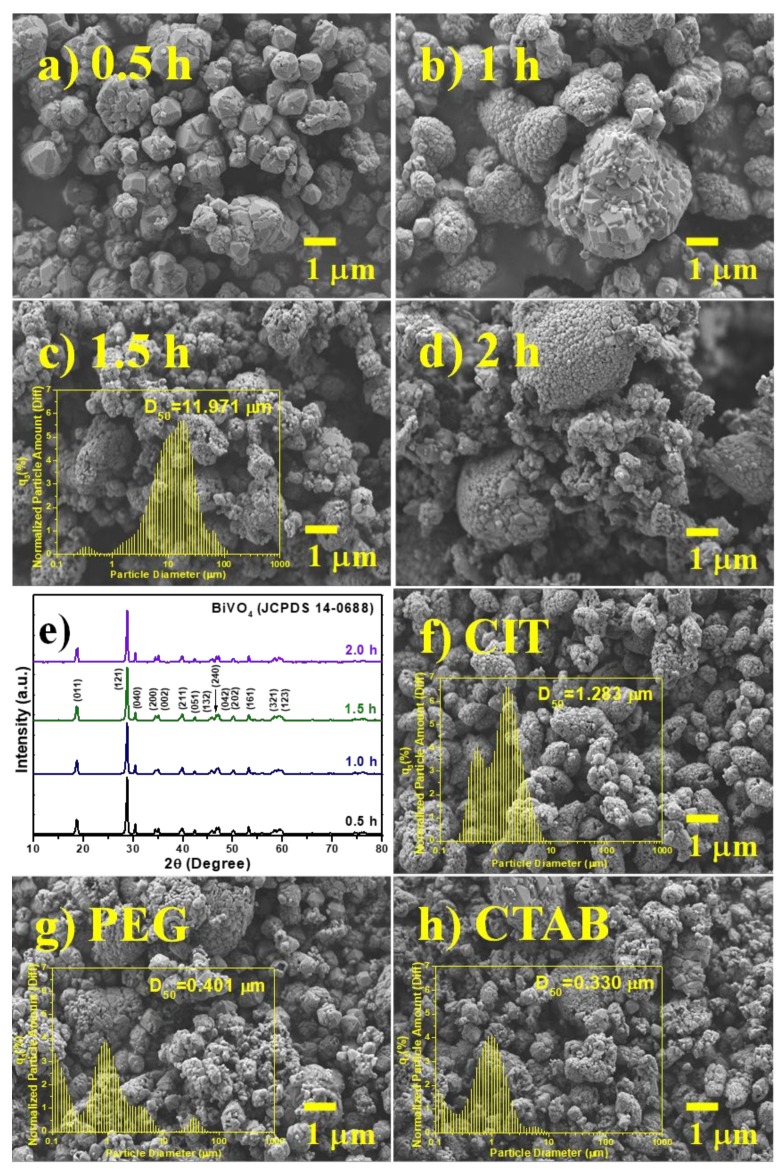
FESEM images (a–d), and XRD patterns (e) of BiVO_4_ photocatalysts with different ultrasound irradiation time (without surfactant). FESEM images (f–h) of BiVO_4_ materials via various surfactants at 1 wt%. Insets reveal the particle size distribution for the corresponding samples.
